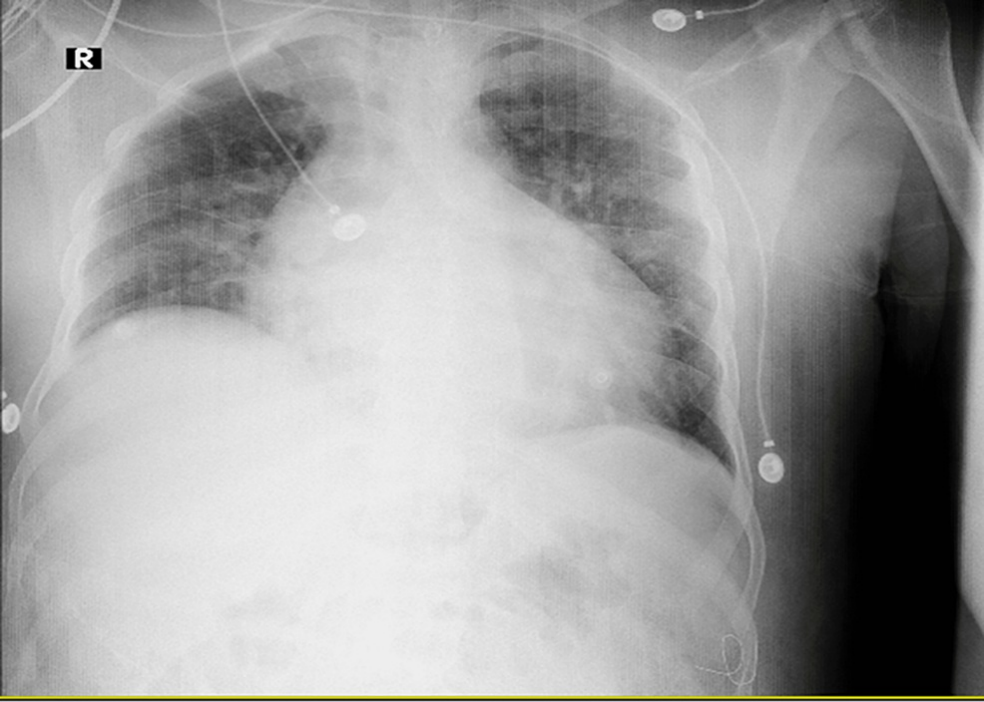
Chest X-ray showing enlarged cardiac silhouette
